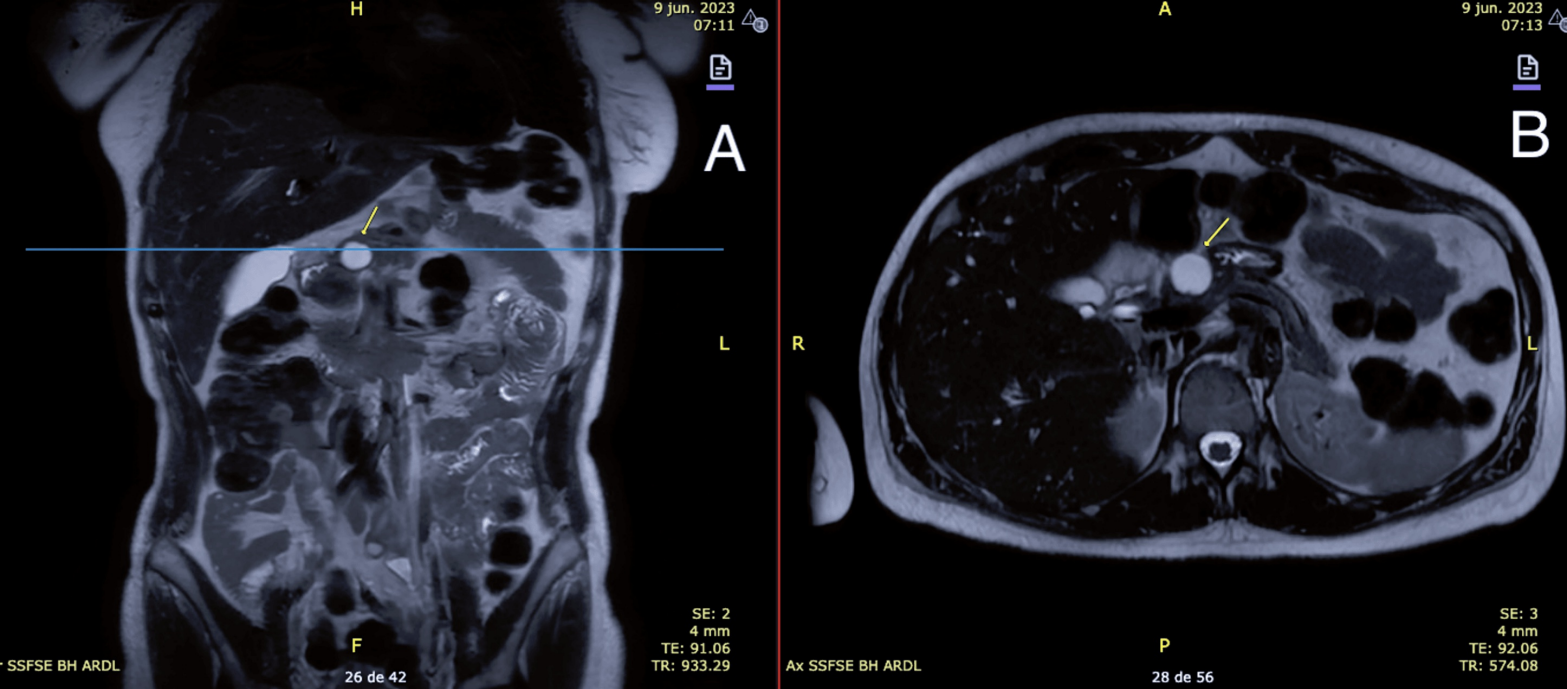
Magnetic resonance imaging (MRI): (A) coronal plane and (B) axial plane Cystic lesion in the pancreatic neck, without aggressive features, measuring 20 mm in its maximum diameter, with no clear communication with the main pancreatic duct. No other focal lesions are identified. The main pancreatic duct is of normal caliber
